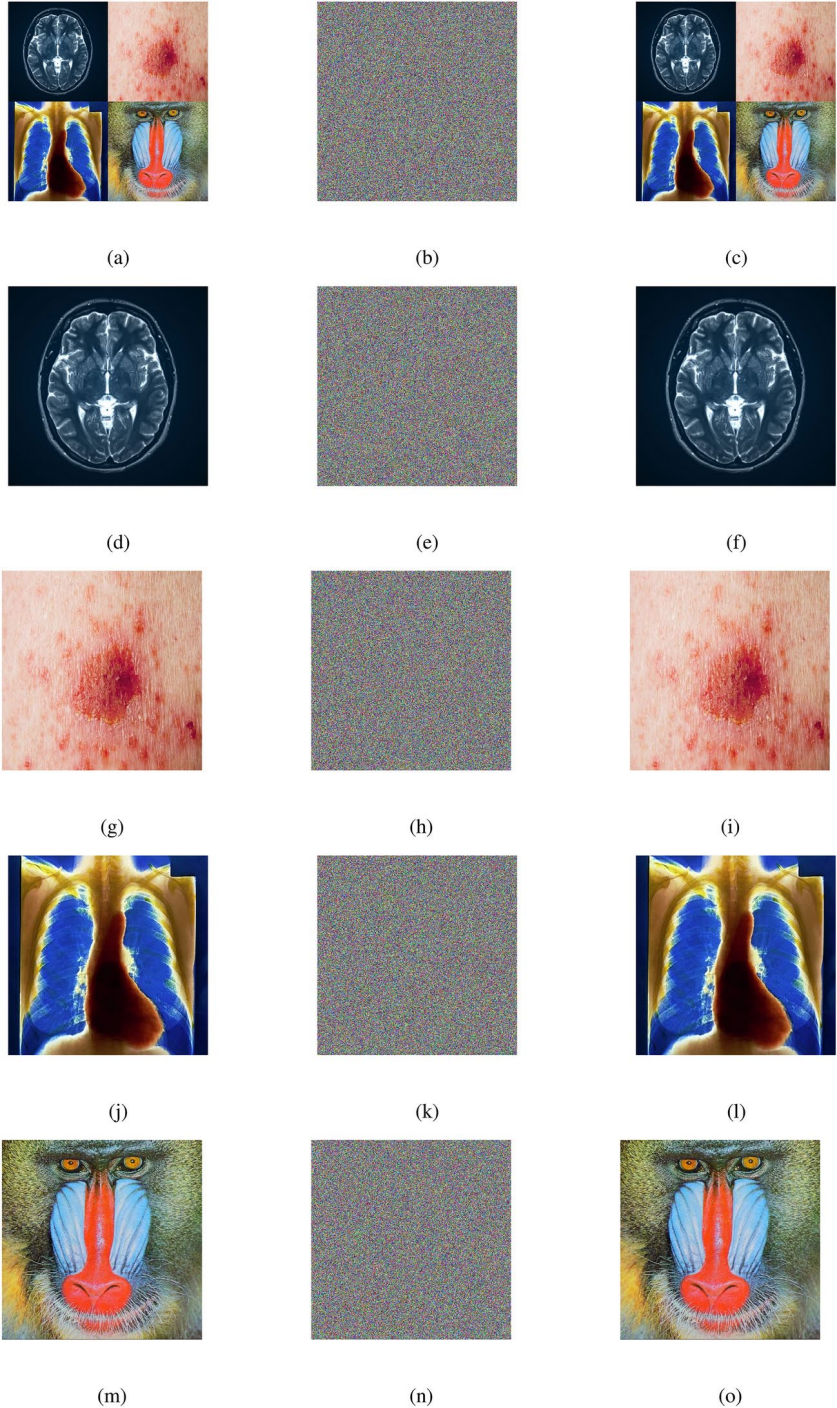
(a–c) Multiple images (original, encrypted, and decrypted), (d–f) brain MRI images (original, encrypted, and decrypted), (g–i) skin cancer images (original, encrypted, and decrypted), (j–l) chest X-ray images (original, encrypted, and decrypted), (m–o) Baboon images (original, encrypted, and decrypted).
